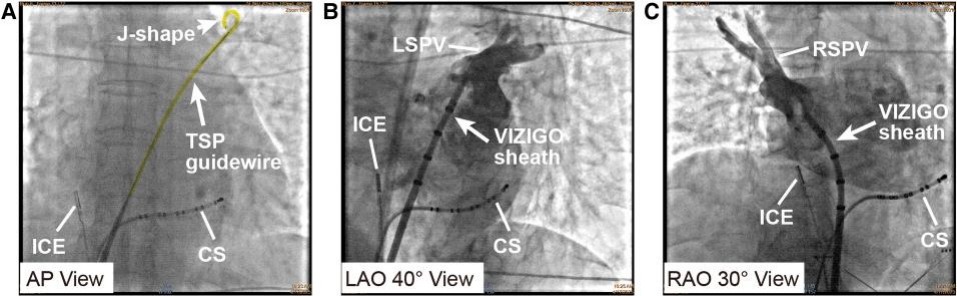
Introducing the sheath into the left atrium. (*A*) The transseptal puncture guidewire (yellow marking and long white arrow) was introduced into the left atrium and reached left superior pulmonary vein (LSPV) safely with a ‘J shape’ (short white arrow). (*B*) Left superior pulmonary vein angiography was performed with VIZIGO sheath (long white arrow) introduced into the left atrium by transseptal puncture wire. (*C*) Right superior pulmonary vein angiography was performed with VIZIGO sheath (long white arrow). CS, coronary sinus catheter; ICE, intracardiac echocardiography; LSPV, left superior pulmonary vein; RSPV, right superior pulmonary vein; TSP, transseptal puncture.
